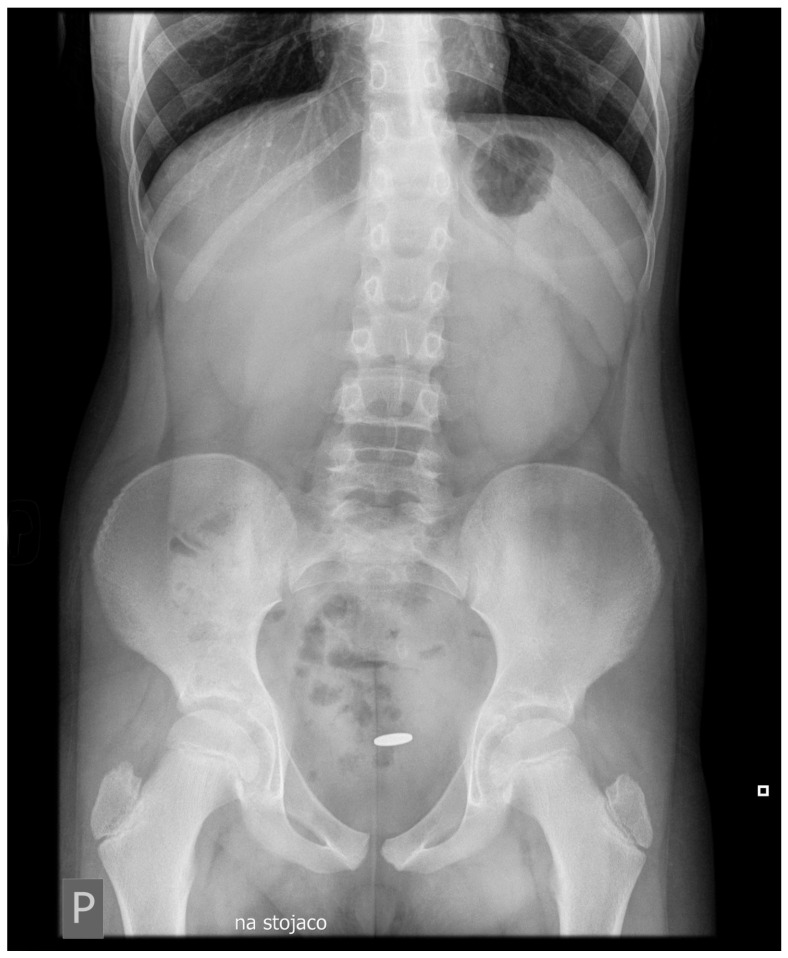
Abdominal X-ray with visualization of the foreign body.
